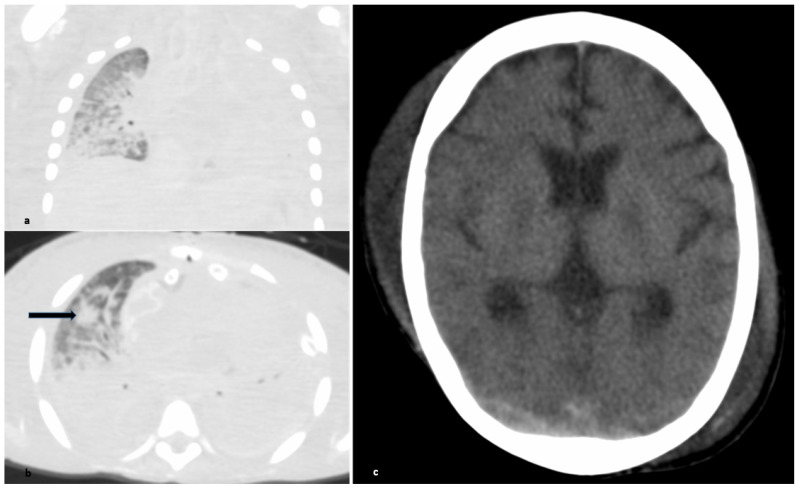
Postmortem computed tomography (PMCT): (a) Coronary CT image (lung section); (b) axial CT image (lung section); patchy area of consolidation ventral (blue arrow); (c) axial CT image (section of head).
